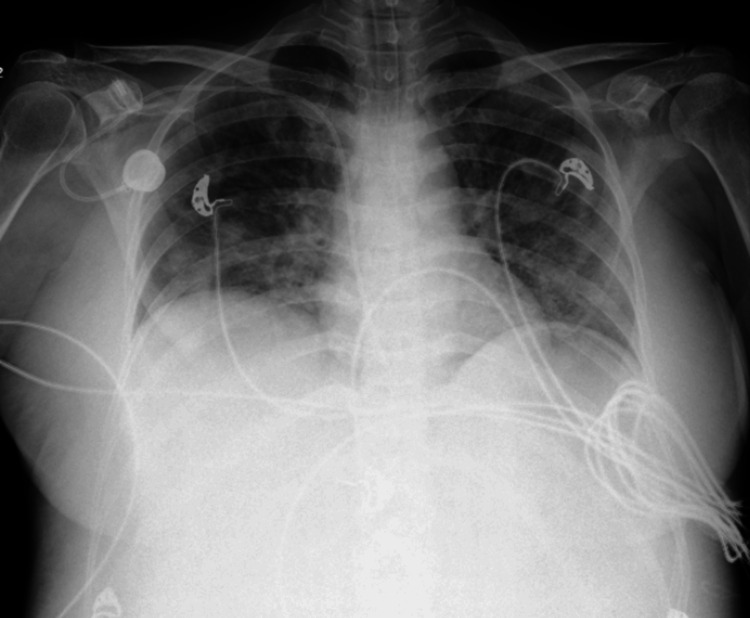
Chest X-ray demonstrating right lower lobe consolidation
